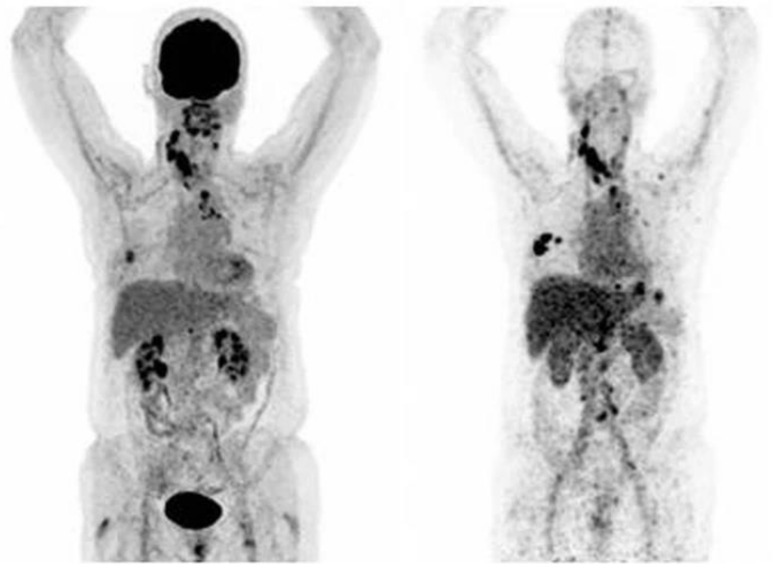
PET-Scan of [18F]FDG (left) and [^89^Zr]Zr-trastuzumab (right) of a patient with a [^89^Zr]Zr-trastuzumab scan considered HER2-positive (Figure adapted from Bensch et al. [53] Creative Commons Attribution 4.0 International License).
